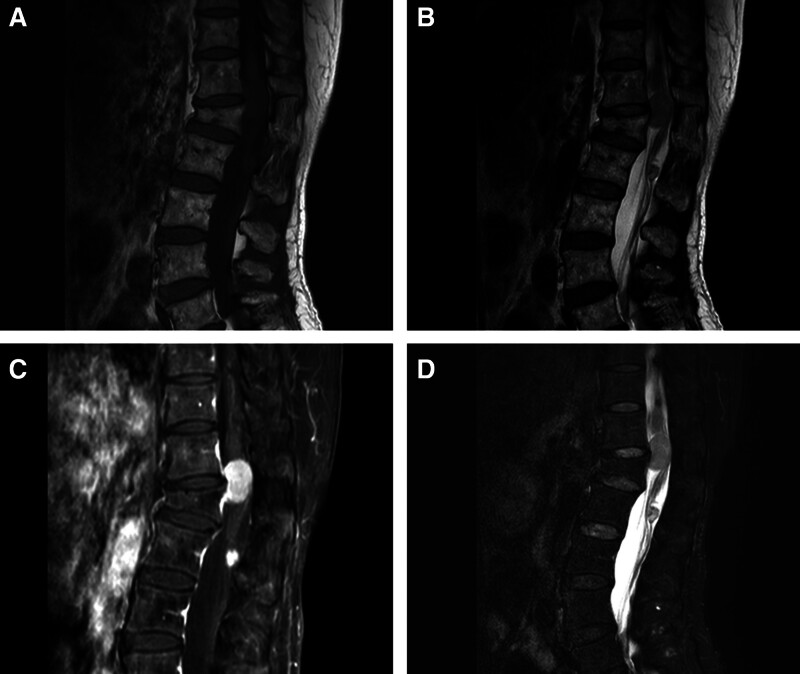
Preoperative sagittal MRI demonstrated 2 intradural extramedullary lesions in thoracic and lumbar levels (A–D).
